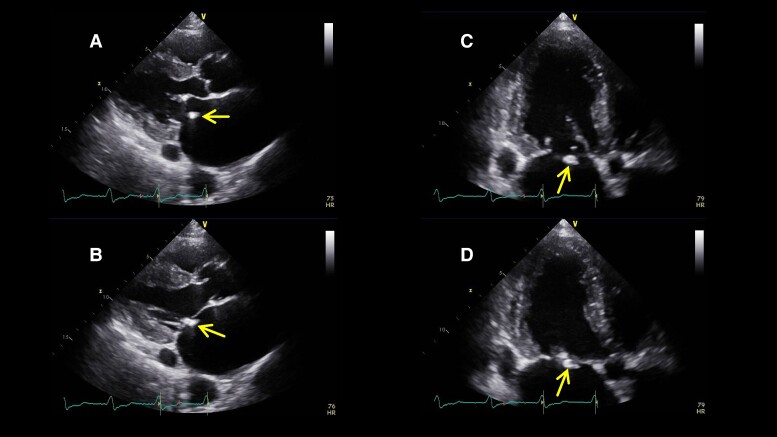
Parasternal long axis view in end-diastole (*A*) and end-systole (*B*) and apical long axis view in end-diastole (*C*) and end-systole (*D*) with the detached annuloplasty ring (arrow) on transthoracic echocardiography.
